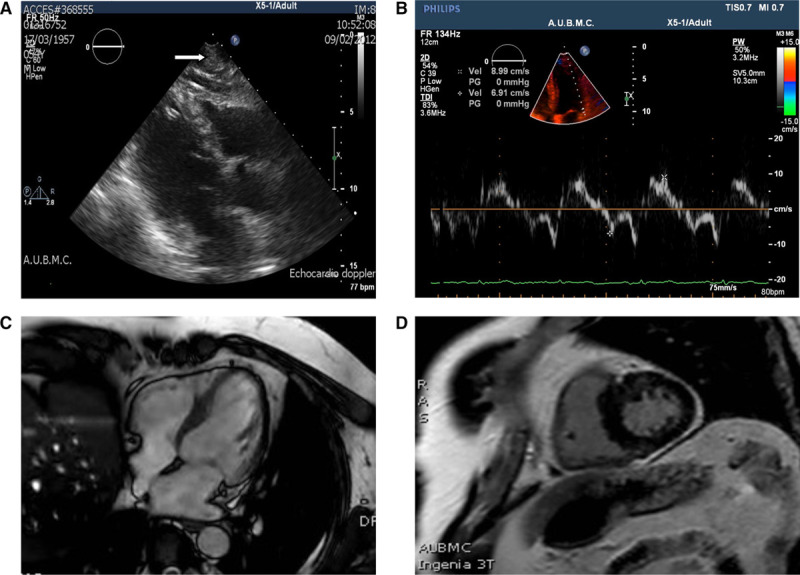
Advanced cardiac imaging of genotype- and phenotype-positive patient. The 48-y-old man (patient II-1 from DH232-A) is symptomatic, carries the p.Arg21Cys mutation, and has known left ventricular hypertrophy on echocardiography (arrow; A). Doppler tissue myocardial velocities at the lateral aspect of the mitral annulus (B) show reduced Ea and S velocities, suggesting early systolic and diastolic myocardial dysfunction. Cardiac magnetic resonance imaging (C and D) shows septal hypertrophy (13–14 vs 7 mm) at the lateral wall and mild-to-moderate focal enhancement in the mid-anteroseptum consistent with scarring.
